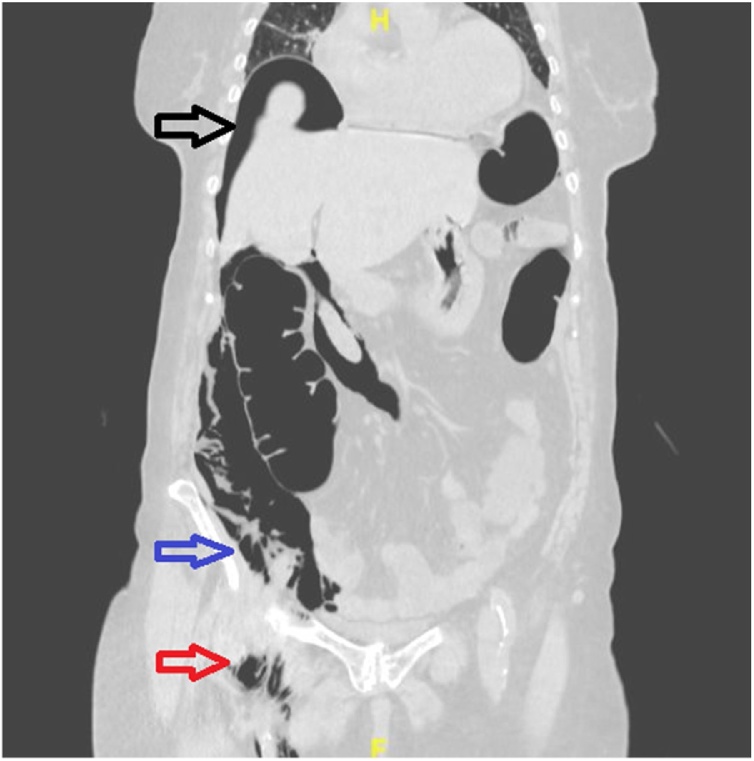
Intraperitoneal (IPP) and retroperitoneal (RPP) presence of air following colonoscopy. Sagittal view CT scans abdomen immediately following colonoscopy confirms massive intraperitoneal (subdiaphragmatic) air (Black arrow), retroperitoneal area (Blue air), and right groin air (Red arrow).
